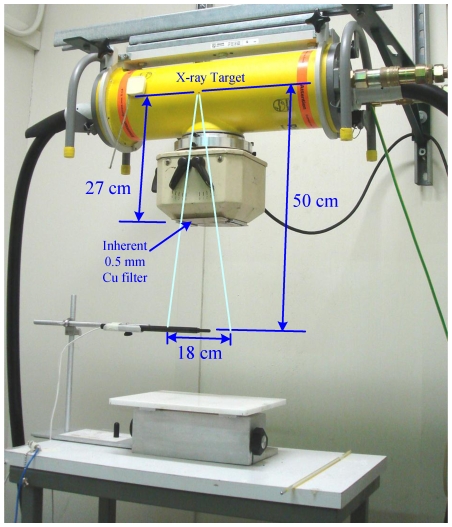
X-ray tube and experimental setup for beam calibration and mammalian cell protection studies. For cell protection studies, 6-well plates with CHO cells were placed in 18×18 cm field where the ionization chamber is located in the figure.
